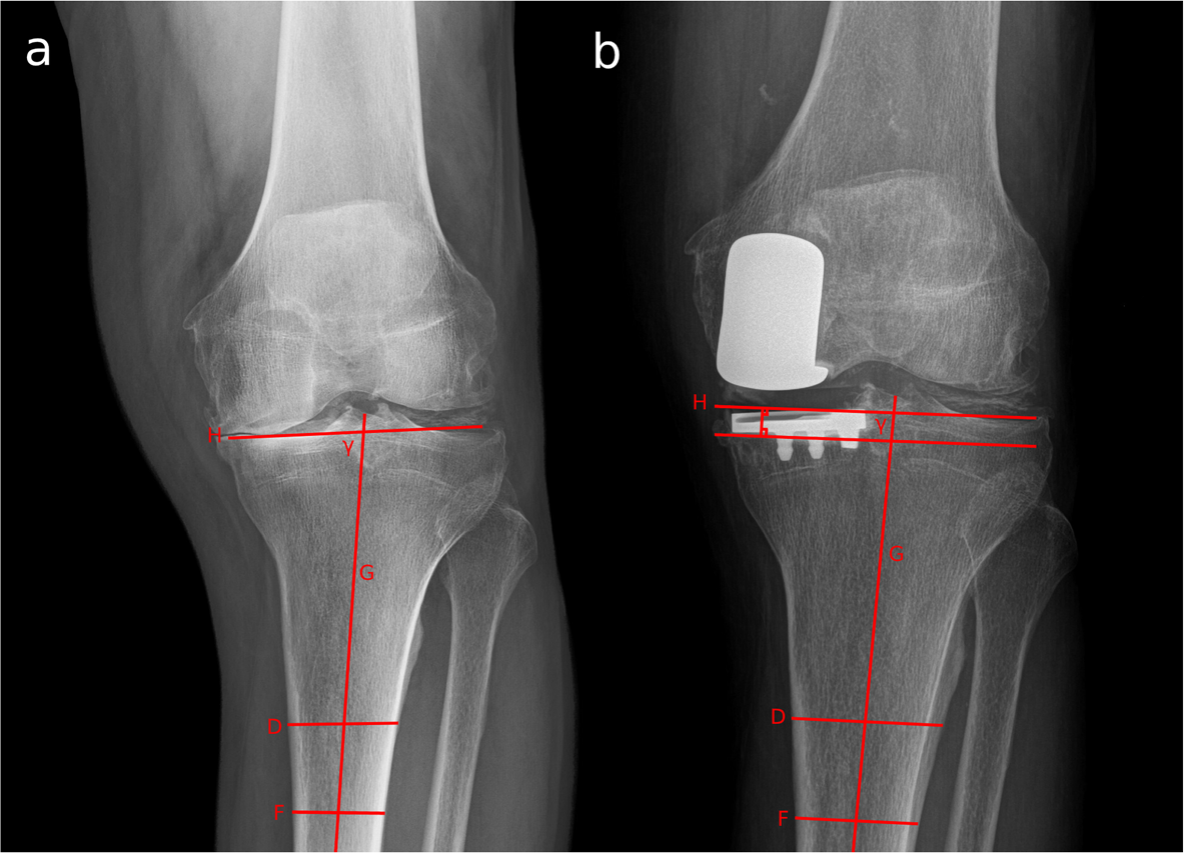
Anteroposterior Knee X-rays of a left knee showing the tibial resection measure method. The angle (gamma) is obtained in the pre-operative x ray (a) between a line (H) that goes through both tibial plateaus and a line that represents the tibial anatomical axis (G), obtained by the center of two lines (D and F) at 7 and 10 cm distal to the joint line. Then the angle is reproduced in the post-operative x ray (b) with the tibial anatomical axis (G) and the non-operative tibial plateau. The distance from the line H to the base of the implant represents the tibial resection
